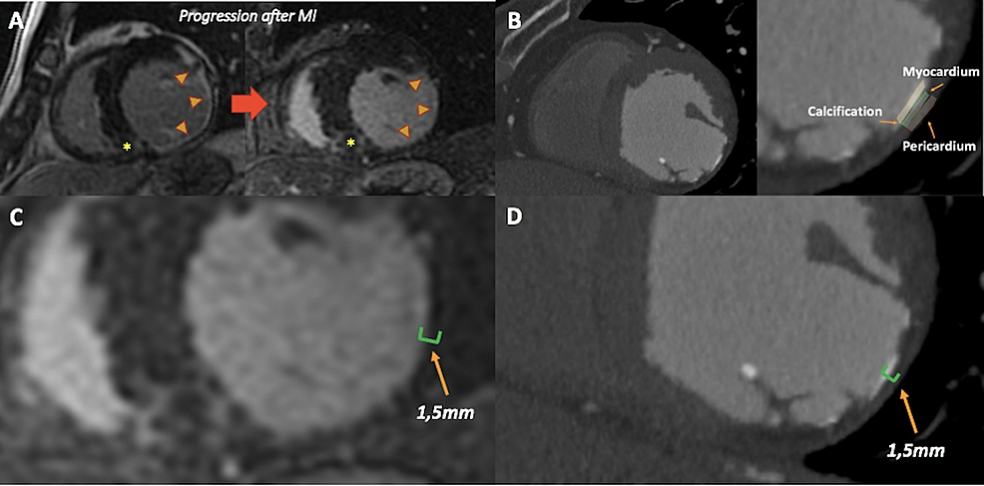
CMR and CT findings A: cardiac magnetic short-axis images showing the progression over 16 years, of the evolution of an extensive myocardial infarction with subendocardial LGE distribution (orange arrows), and a non-ischemic patchy intramyocardial LGE at the basal inferoseptal level (yellow asterisks). B: CCTA short-axis images at the same level confirming the dystrophic calcification of a part of the myocardium at the subendocardial level. C and D: comparative CMR and CCTA assessment of myocardial involvement in short-axis left ventricular views MI: myocardial infarction; LGE: late gadolinium enhancement; CCTA: cardiac computed tomography angiography; CMR: cardiac magnetic resonance
